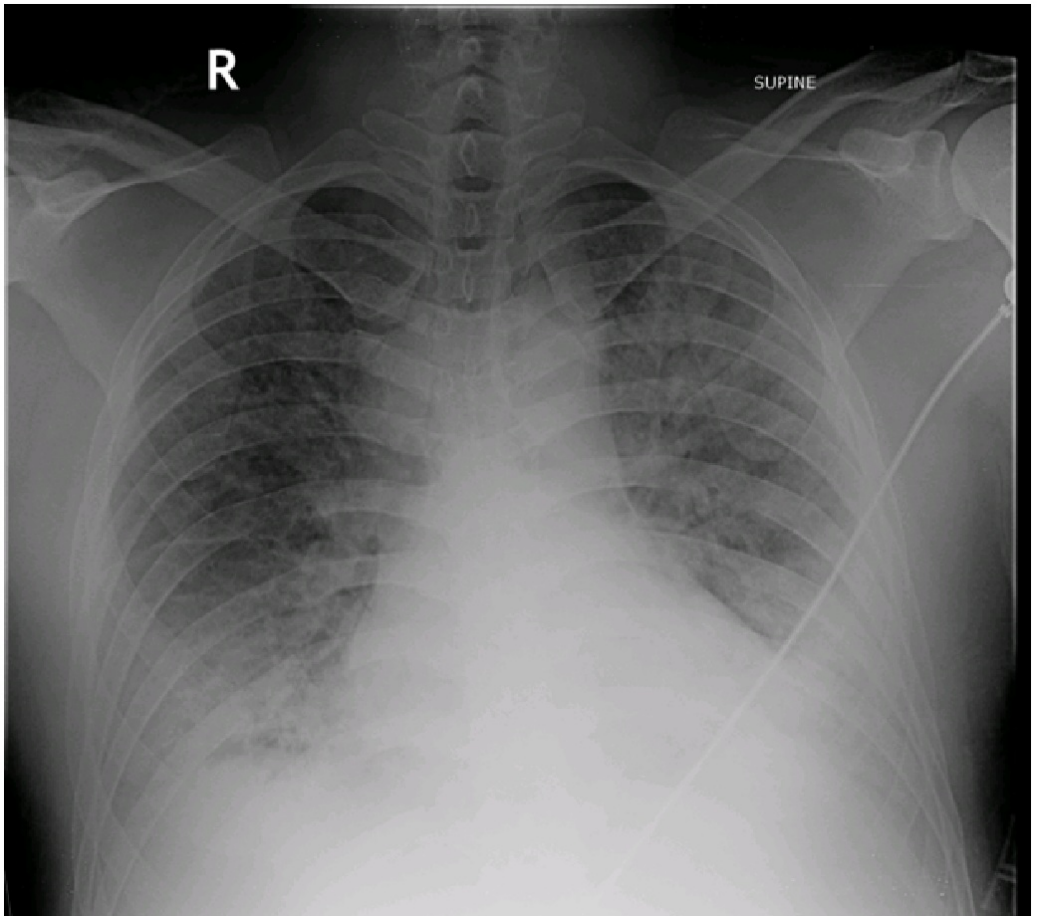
Chest Xray shows bilateral lower lobe infiltrates consistent with non-cardiac pulmonary edema
